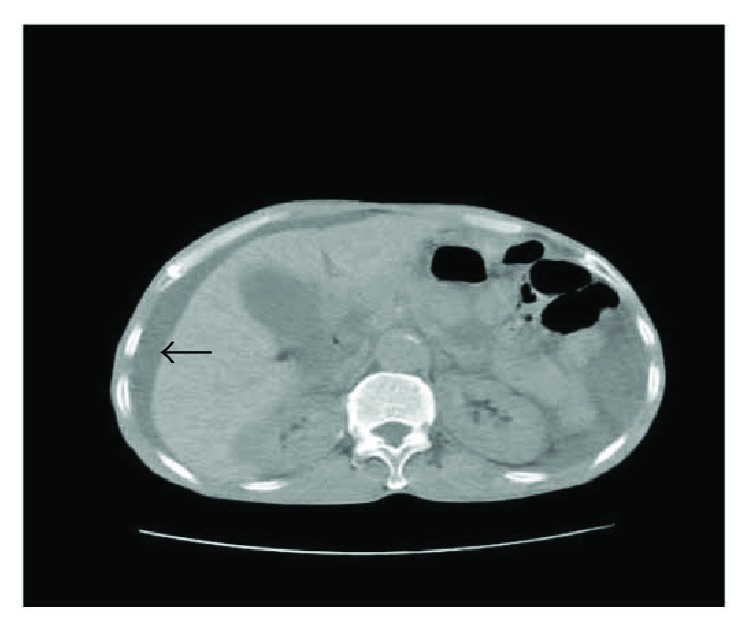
Computed tomography scan of the abdomen showing a large quantity of ascites (black arrow) around the liver.
